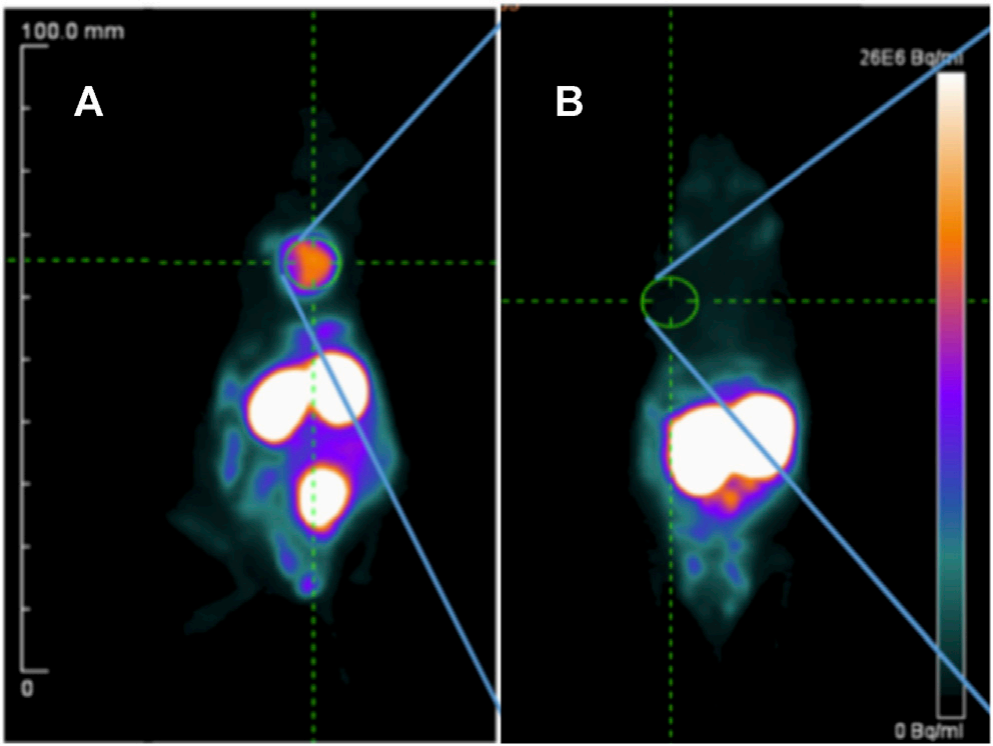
PET imaging of ^68^Ga-PSMA-11 in MDA-MB-231 (A) and MCF-7 (B) xenografts at 30 min post-injection (Morgenroth et al., 2019).
